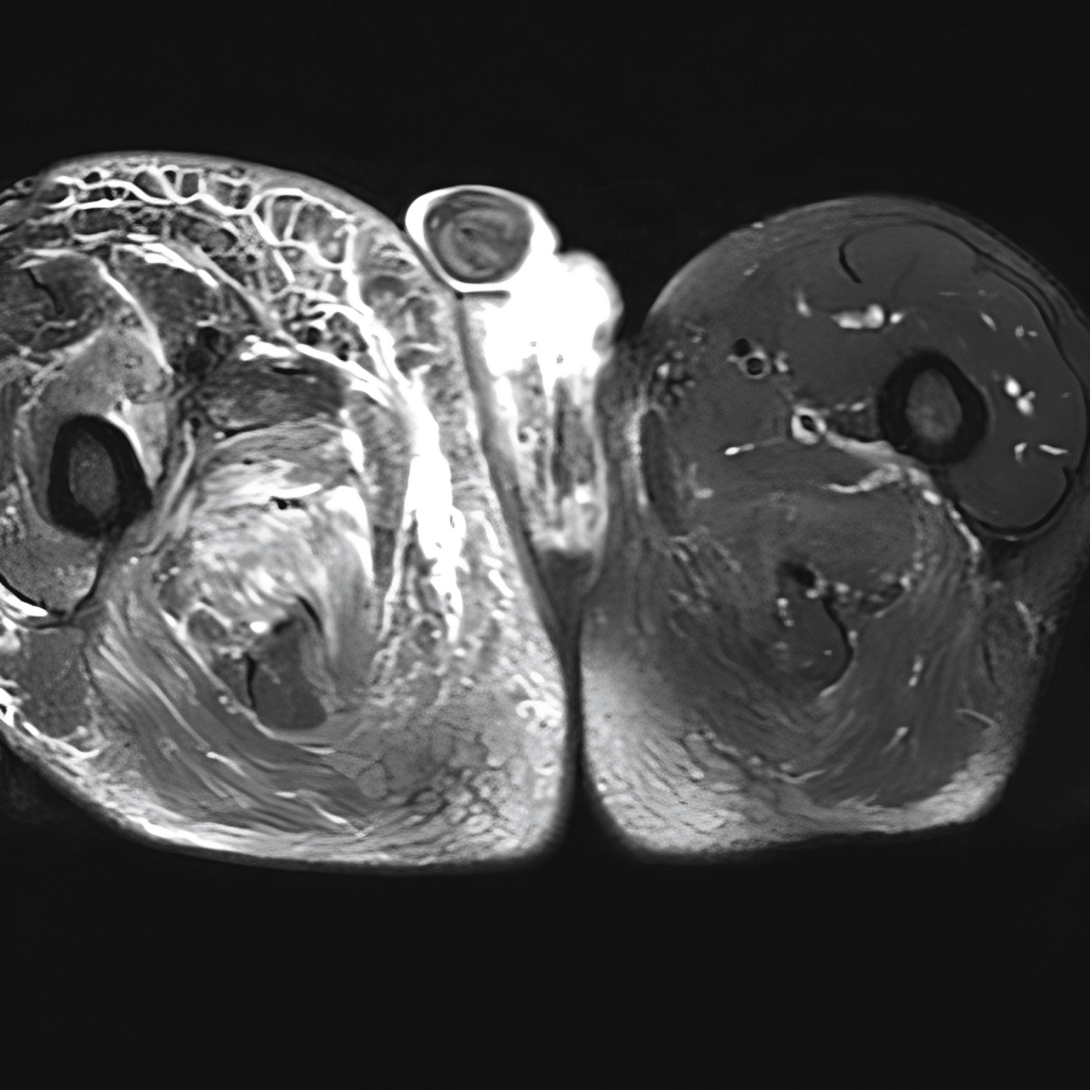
Axial T2-weighted fat-suppressed MRI of the thighs demonstrating extensive hyperintense signal in the subcutaneous and deep soft tissue of the right thigh, consistent with edema and inflammation. There is associated fascial thickening. Note the asymmetry between the left and right thighs, with diffuse tissue swelling in the affected limb.
